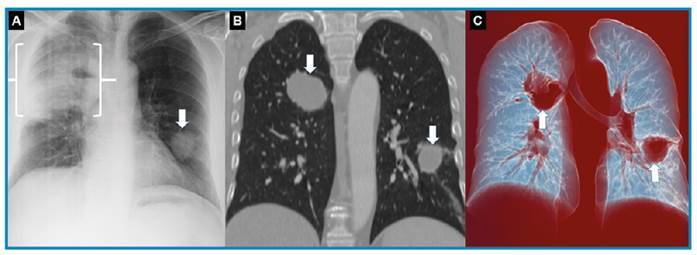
Direct radiography (A) showing an area of consolidated pneumonia (bracket) in the right upper zone and a smooth-edged lesion in the left lower zone (arrow). In the parenchymal, the coronal reformatted CT scan (B) and air-volume rendering 3D reformation sections (C) showing round-shaped lesions in the lower lobe of the left lung and upper lobe of the right lung (arrows).
